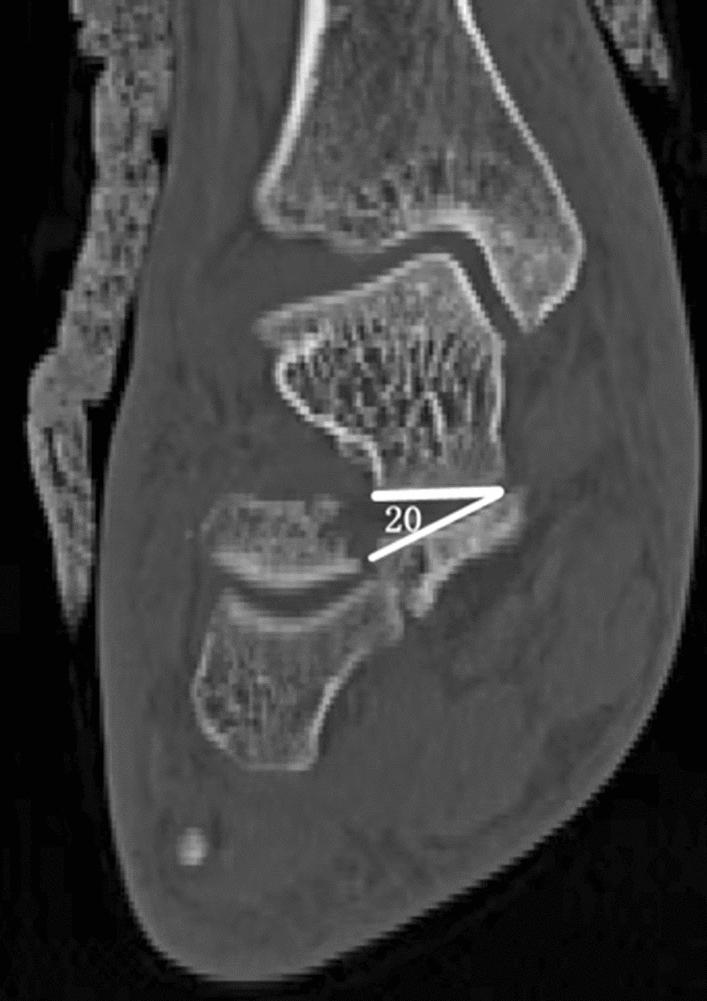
Coronal CT showings the angulation of the sustentaculum fragment.
